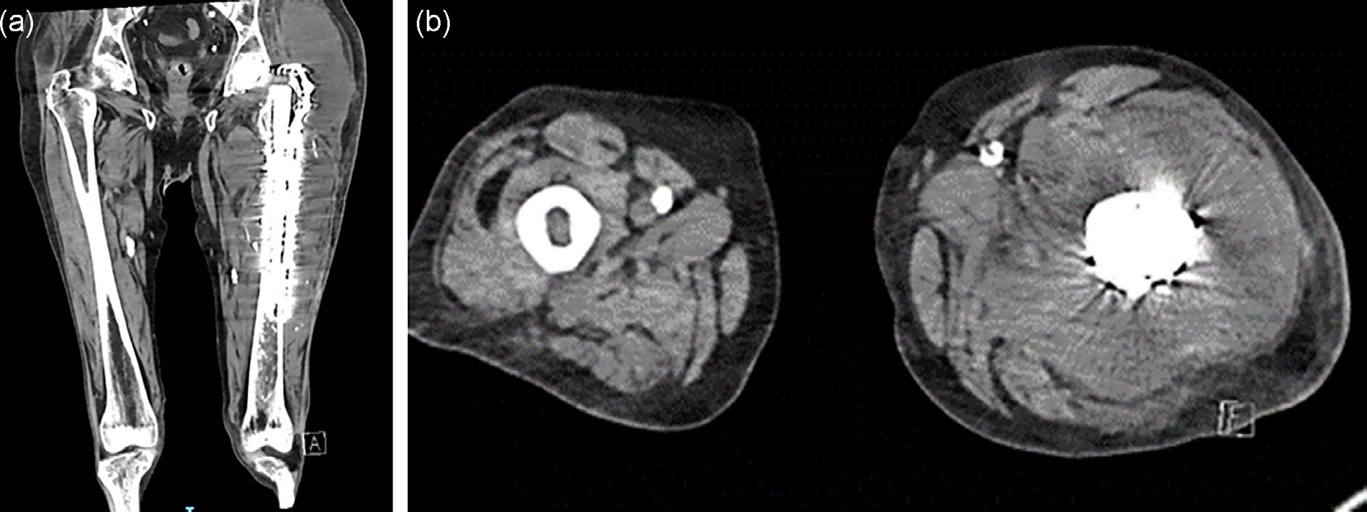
(a) Coronial and (b) axial CT images showing large fluid collection surrounding femoral stem and fixation.
